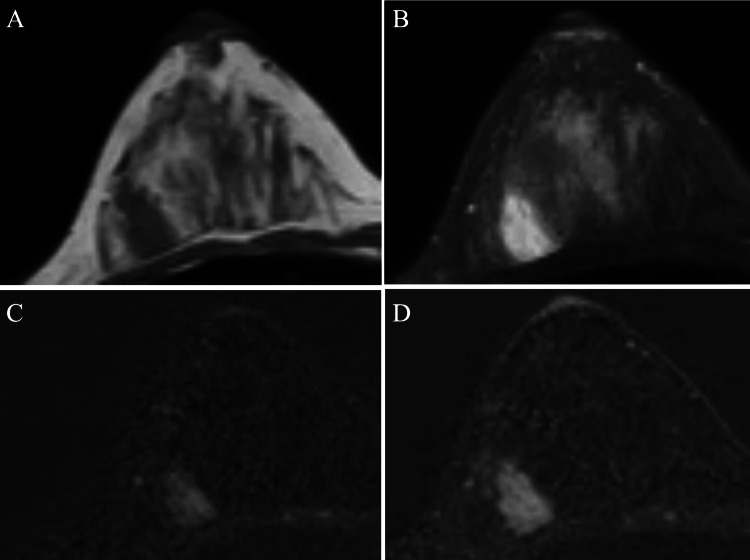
MRI findings MRI showed a well-circumscribed mass on T1-weighted (A), T2-weighted (B), and subtraction (C and D) images. In addition, subtraction images showed a homogenous and persistent enhancement pattern of the tumor (C and D). MRI: magnetic resonance imaging
